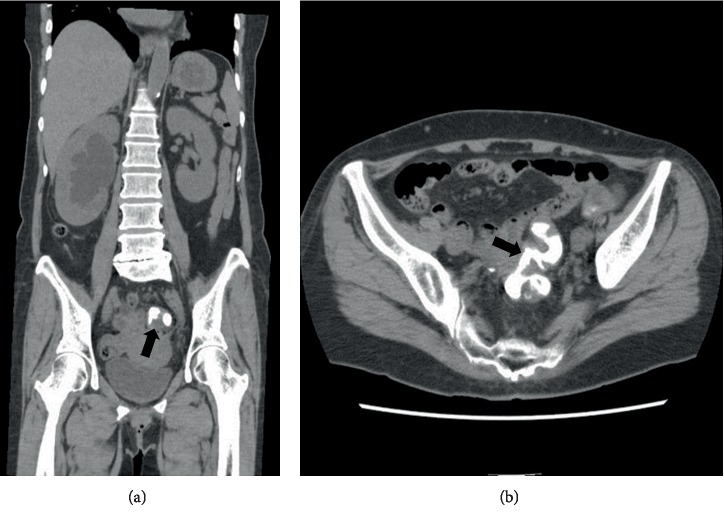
Coronal (a) and axial (b) scan of pelvic CT after retrograde pyelography. Contrast used for retrograde pyelography remains in the sigmoid colon (arrow).
